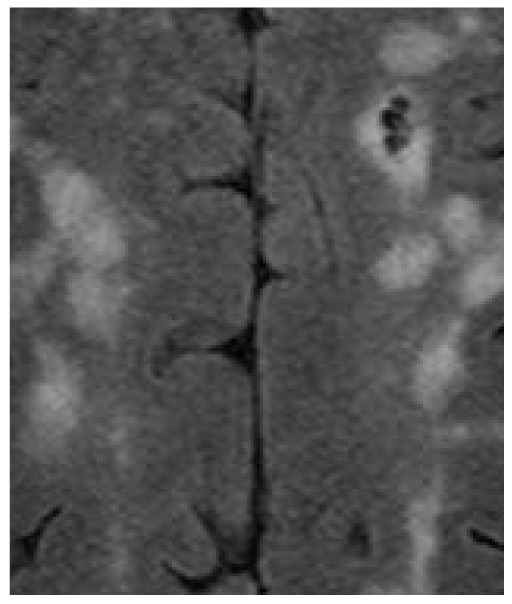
Image showing part of an MRI of the brain: bright patches are the white matter hyperintensities or lesions of the brain, and other parts are brain tissues and fluid.
